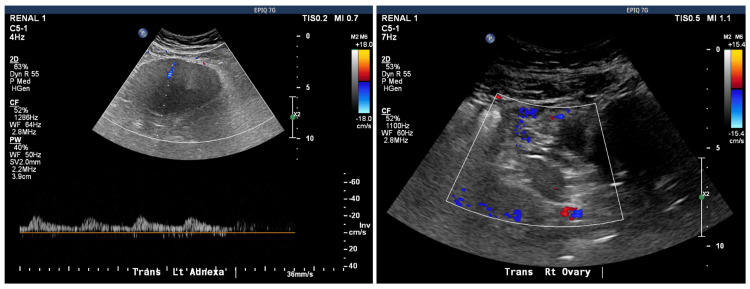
Transabdominal pelvic ultrasound showing no flow to the left adnexal mass versus flow to the right ovary
